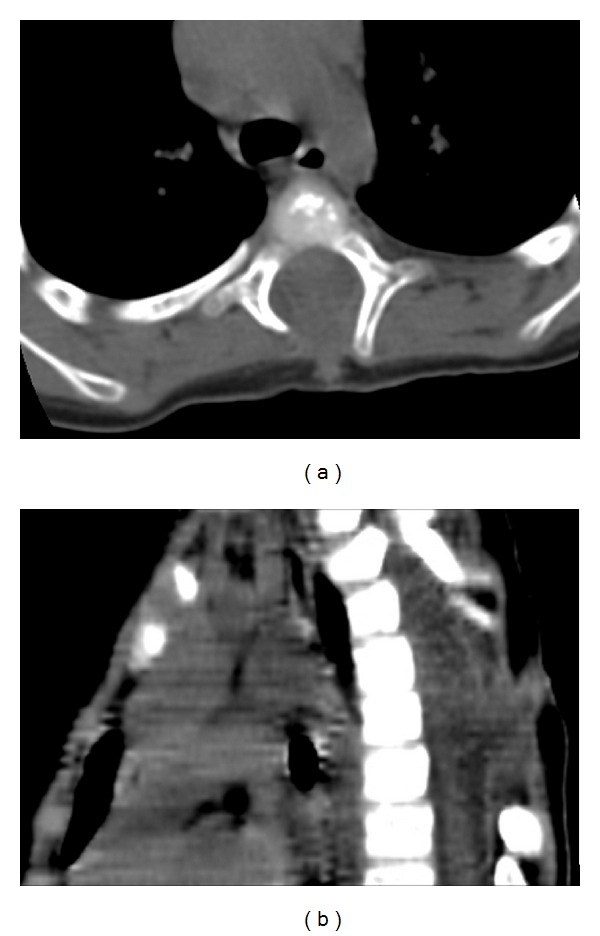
Axial (a) and sagittal (b) CT images. There is deficiency of the posterior elements at T3–T6, with remodeling and widening of the spinal canal. A soft tissue attenuation tract extends to the skin surface.
